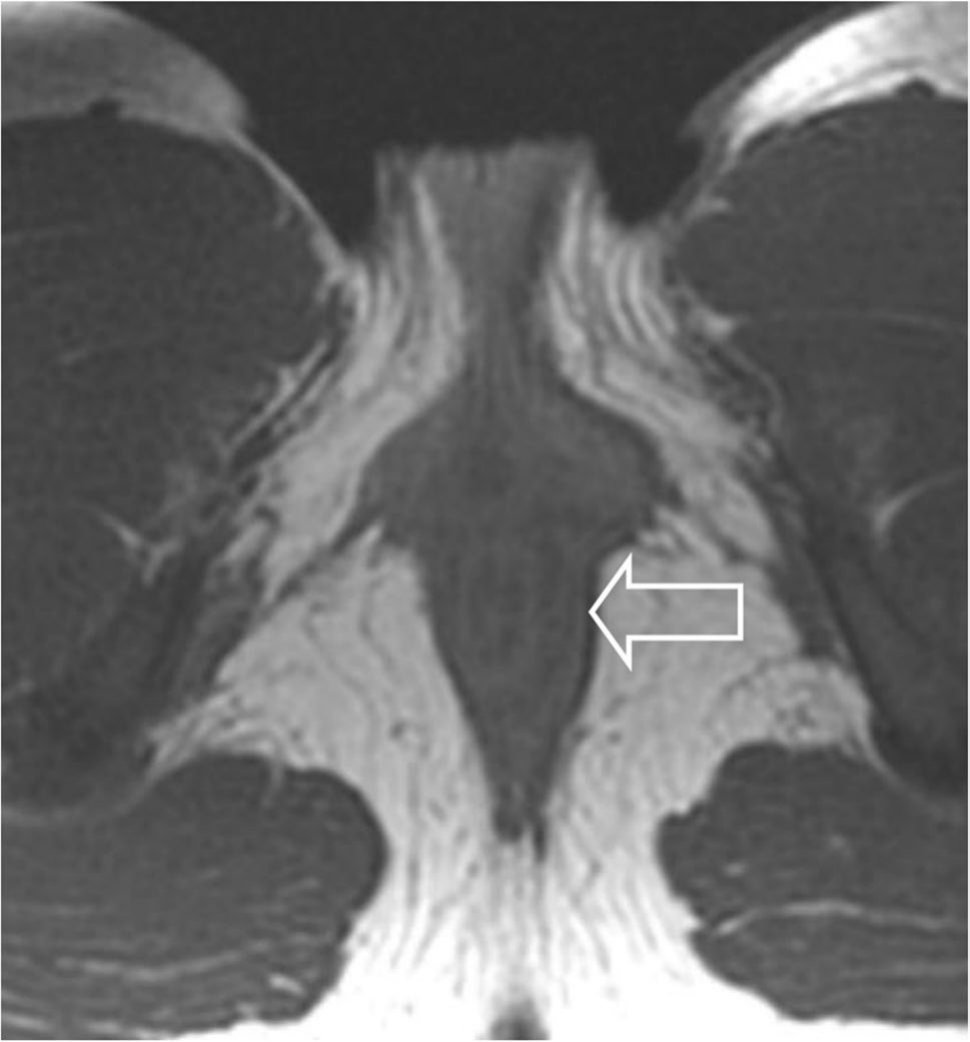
Axial T1 response assessment magnetic resonance image of a 14-year-old girl (patient 2) after two cycles of chemotherapy (due to optimal response after two rather than the standard three cycles) shows minimal residual local disease (*arrow*)
